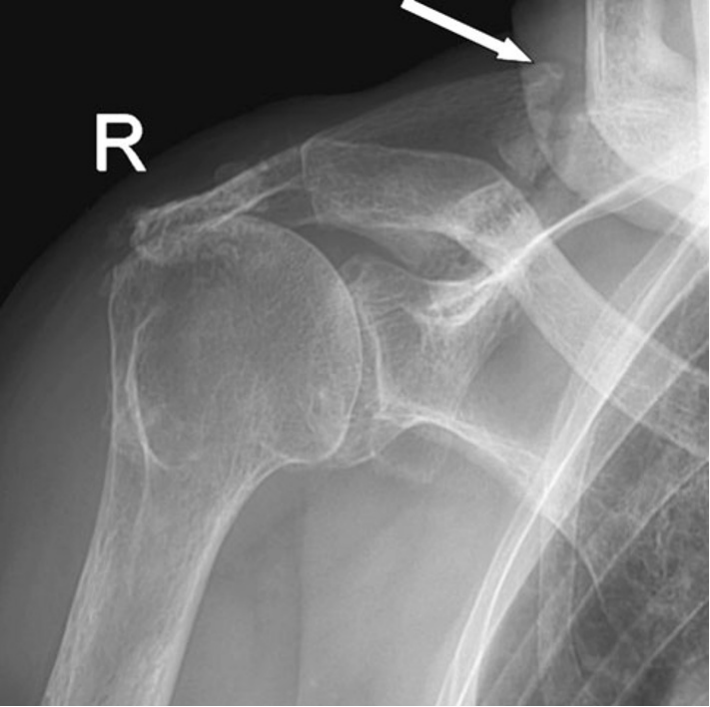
(Patient 2) X-ray of right shoulder showing fracture of the right scapular spine with callus formation but no consolidation 3 months after initial symptoms
